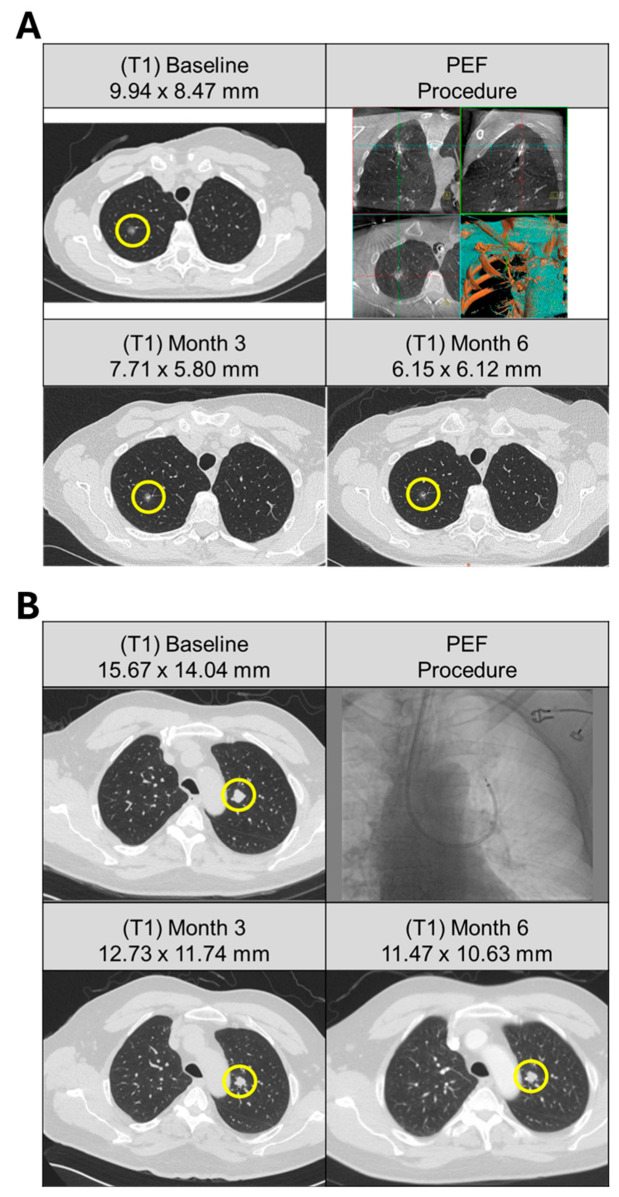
Pulmonary metastases from solitary and multifocal patients treated with bronchoscopic ablation. (A) Patient (A25) with history of breast and bladder cancer, presented with solitary right upper lobe lesion with no identifiable primary source. (Top left) CT scan of 9.9 mm nodule at baseline (yellow circle). Three activations were delivered to the target lesion via bronchoscopic access (top right). (Bottom row) Response of the target lesion at 3 and 6 months following ablation demonstrated a progressive decrease in size, measuring 7.7 mm and 6.2 mm, respectively. (B) Patient (A02) presented with multifocal solid pulmonary nodules subsequently deemed metastatic papillary thyroid carcinoma with cervical lymph node involvement. Five pulmonary lesions were identified at baseline (top left). Ablation was performed on a single-target lesion (yellow circle) in the left upper lobe using a bronchoscopic approach (top right) with nine activations. (Bottom row) Response of the target lesion at 3 and 6 months following ablation demonstrated size stability, measuring 12.7 mm and 11.5 mm, respectively.
